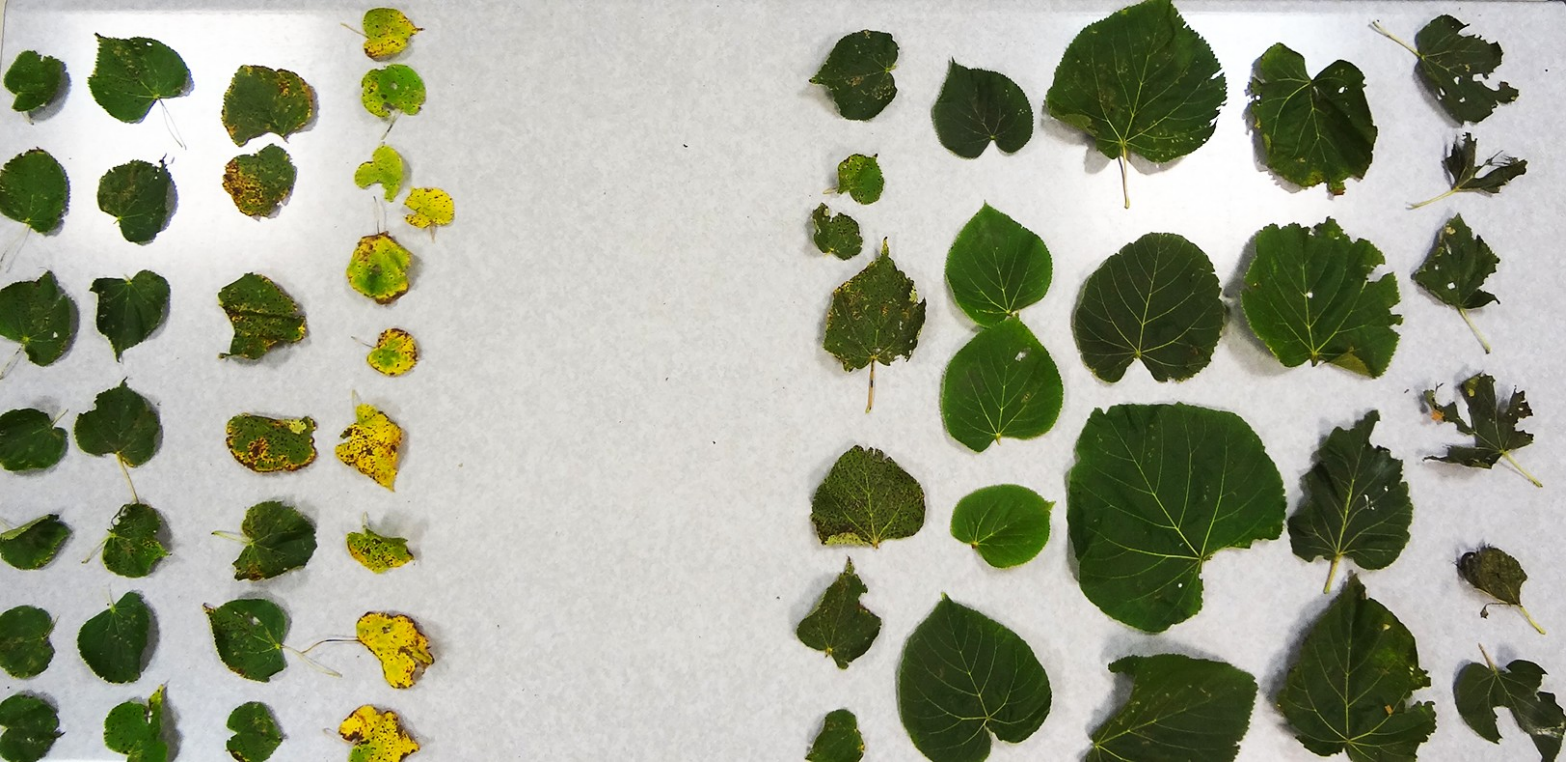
Leaves collected from CT, on the left, leaves from PT from within the offshoots’ zone, on the right, September 30, 2016. Photo by M. Suchocka.
